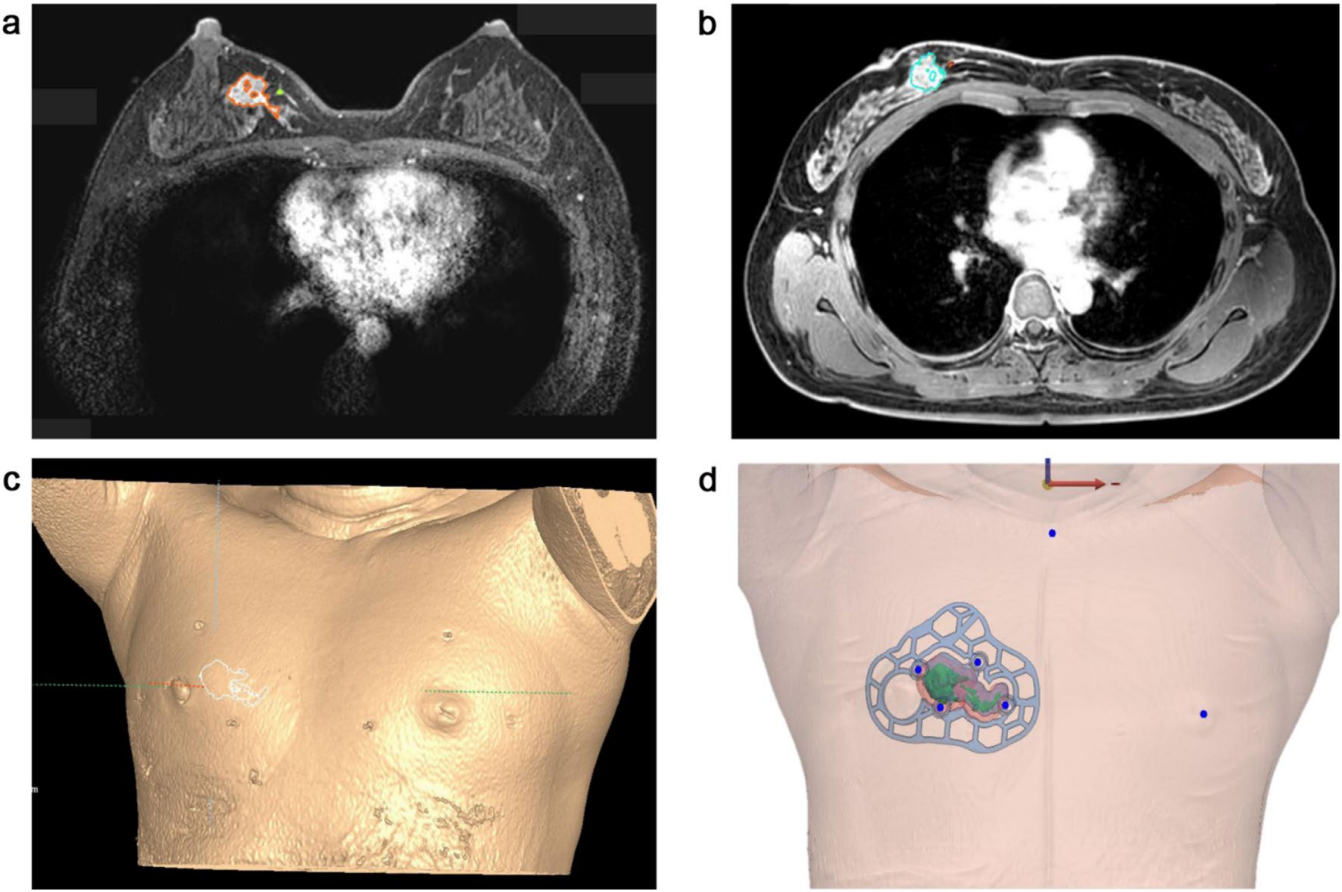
Segmentation of the breast and tumor in prone/supine magnetic resonance imaging (MRI) for breast surgical guide (BSG) modeling. (a) Segmented tumor on prone MRI, (b) Segmented tumor on supine MRI, (c) 3D modeling of the breast and tumor on supine MRI, (d) BSG tailored to the patient's breast surface to target tumors inside the breast.
